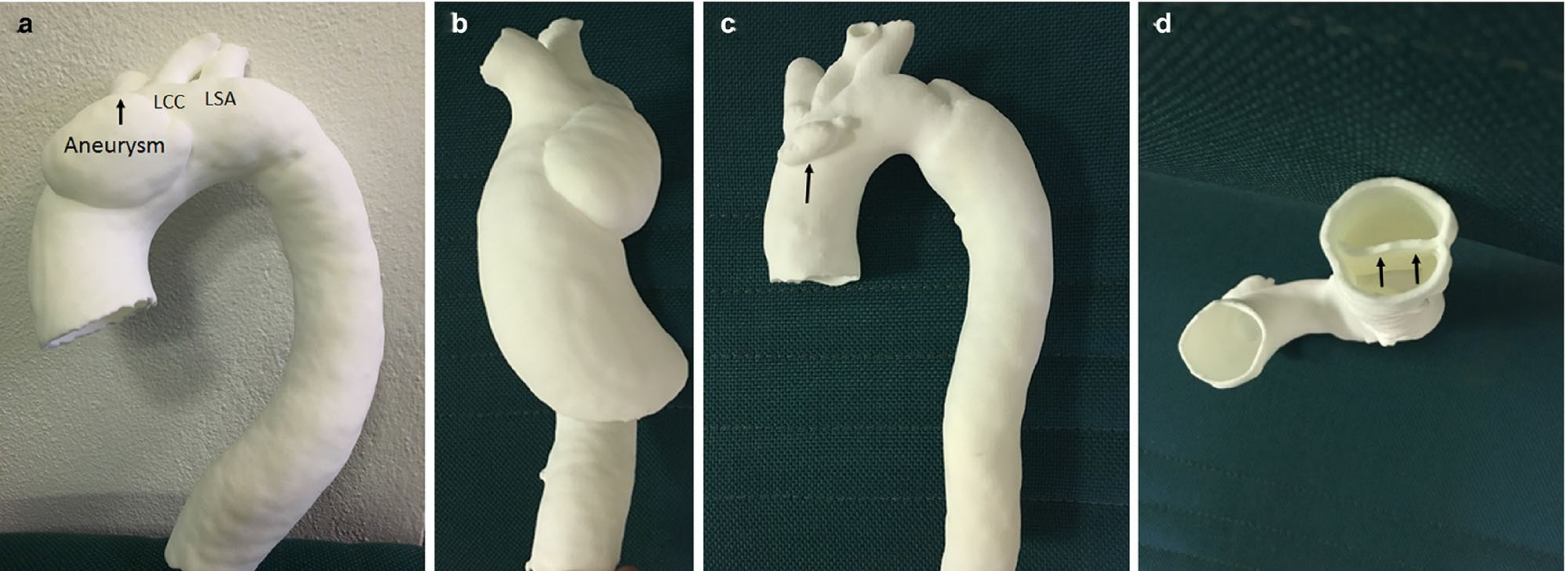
3D printed models generated from cardiac CT images which shows the Aortic Aneurysm structure from different angles. a Model shows the aortic aneurysm in relation to the three arterial branches starting from the aortic arch, namely left subclavian artery, left common carotid artery and innominate artery (arrow). b Lateral view showing the aneurysm. c Anterior view with artifact (arrow) in the aortic arch due to image post-processing. d Caudocranial view of aortic dissection showing intimal flap (arrows) [28].
